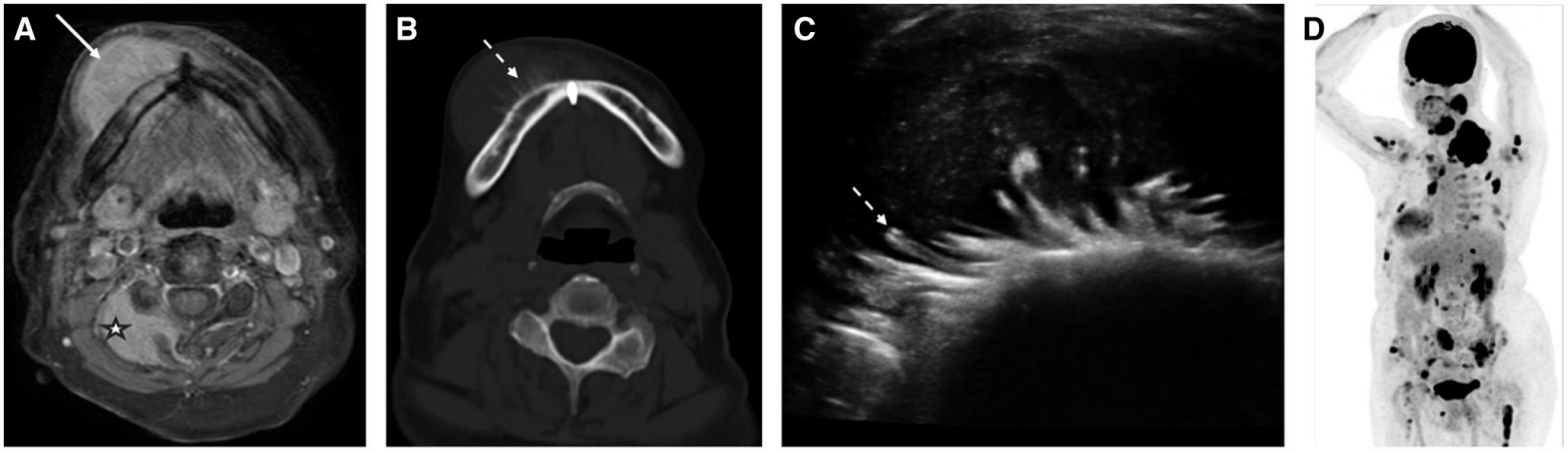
EMP of the right mandible. (A) Axial fat-suppressed post-contrast T1-weighted MRI shows a homogeneously enhancing lesion arising from the infiltrated right mandible (white arrow). There is further EMP infiltrating the right paraspinal muscles and the C3 vertebral facet and lamina (star). (B) and (C) Axial NECT and US show aggressive sunburst periosteal reaction along the buccal cortex of the right mandible consistent with rapid tumour growth (dotted arrow). The lesion is hypoechoic on US. (D) Maximum intensity projection (MIP) from a whole-body FDG-PET-CT in oblique plane demonstrates multiple FDG-avid MM deposits throughout the body.
